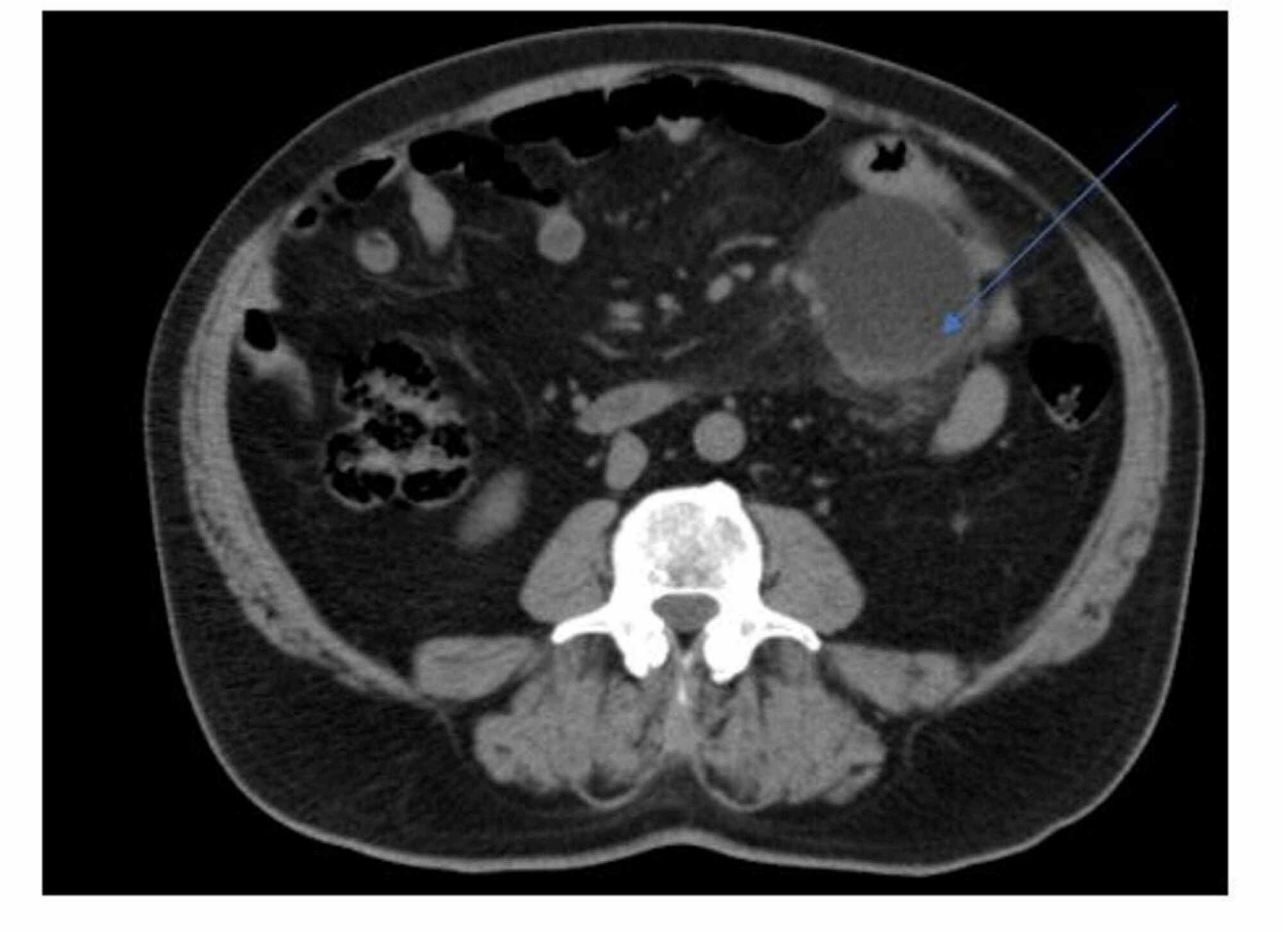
Abdominal CT scan frame Showing a cystic hypodense lesion with hyperdense content located between the mesenteric vessels in the left lower abdominal quadrant, which determines a dislocation of the intestinal loops and vessels along with hyperdensity of the surrounding fatty tissue. CT: computed tomography
